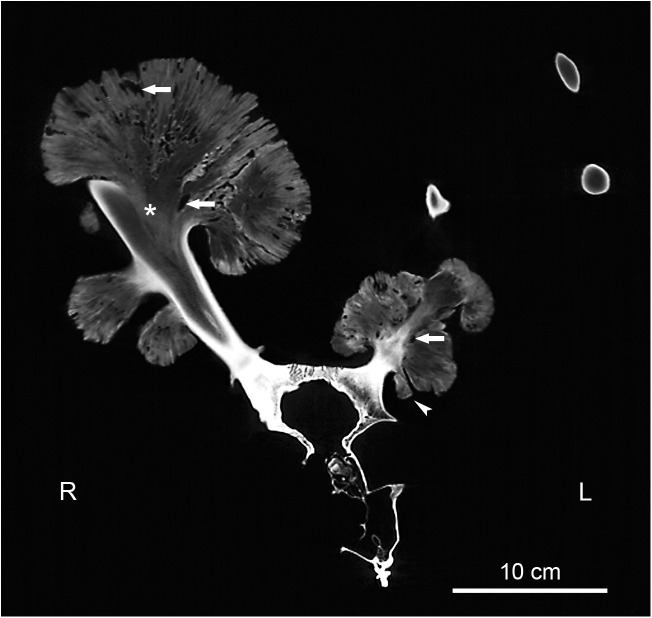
CT image showing the cranium and antlers of the white-tailed buck (*Odocoileus virginianus*). Note the normal composition of the antlers with a compact cortex and a cancellous core, as seen in the right antler and the two virtual cross sections of the distal part of the left antler in the upper right corner of the large image. Also note the continuity of the spongiosa (asterisk) and the cortex (present only in the basal stalk region) of the large exostosis from the right antler with the respective components of the parent bone. The seeming non-continuity of the cancellous bone between the antler and the smaller exostosis from the right antler as well as its seemingly bipartite structure is due to the fact that the slice is not located in the central axis of its stalk. Arrows: radiolucent areas within the outgrowths. R: right side; L: left side.
